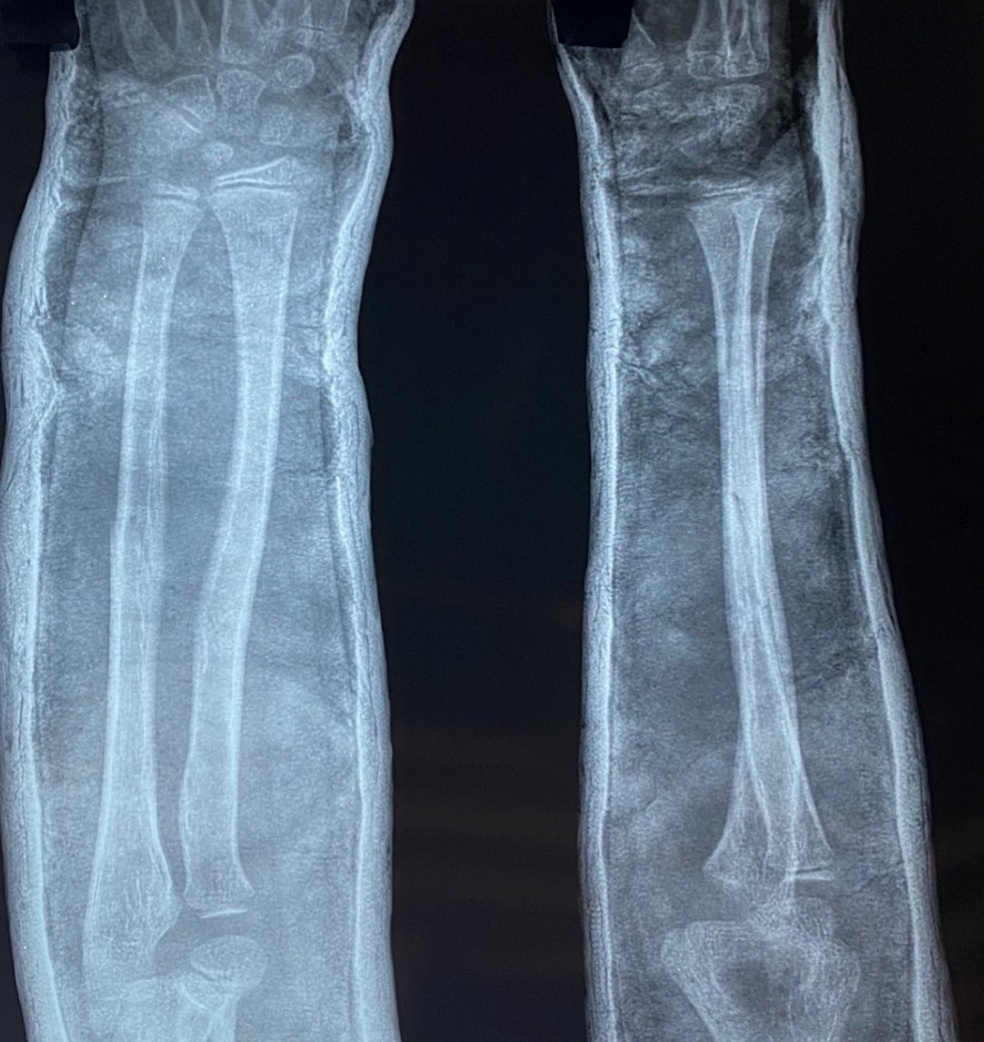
Post-operative radiograph taken two weeks after casting
